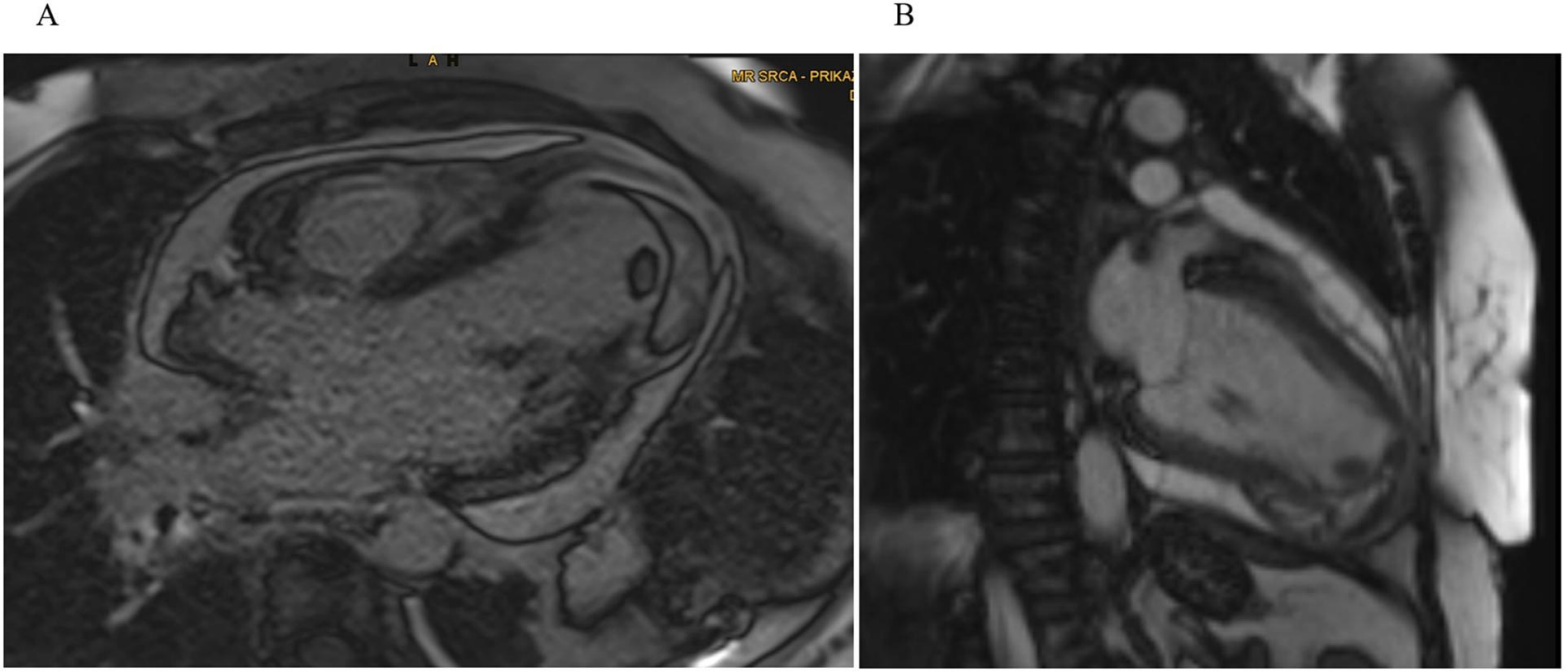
Cardiac MRI. (A) Four-chamber and (B) two-chamber views showing a well-demarcated hypointense lesion within the inferoapical wall extending into the septum, consistent with intramyocardial hemorrhage and a clearly defined intracavitary thrombus visible at the LV apex.
